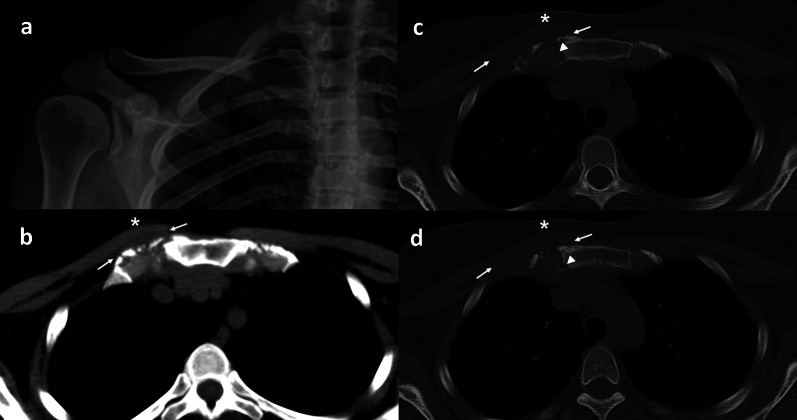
Right first sternochondral psoriatic arthritis in a 36-year-old woman. CT adds valuable information in anatomical complex areas like in this patient with normal X-ray examination of the right shoulder girdle including the right sternoclavicular joint (a). Axial CT images, either on soft tissue windowing (a) or bone algorithm (c, d) show asymmetry (*) and the loss of definition of the surrounding fat planes (thin arrows) between the first sternochondral joint and the muscles can also be appreciated. Small erosions in the sternal side are also visible (arrow heads)
